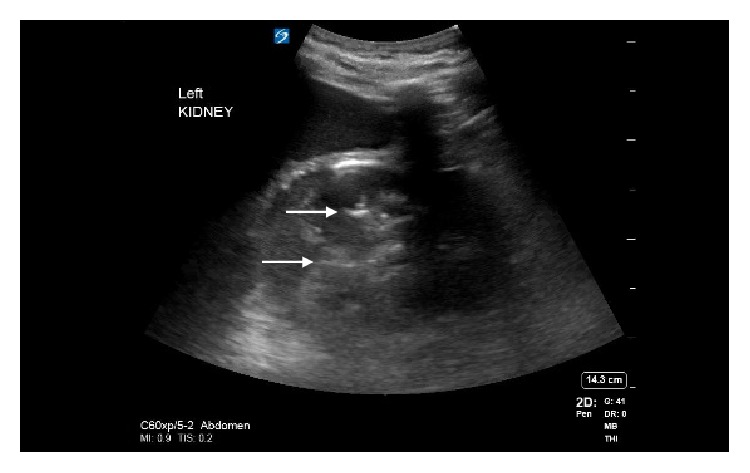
Longitudinal ultrasonography of the left kidney showing a- lines (arrows), indicating free air in the renal parenchyma.
